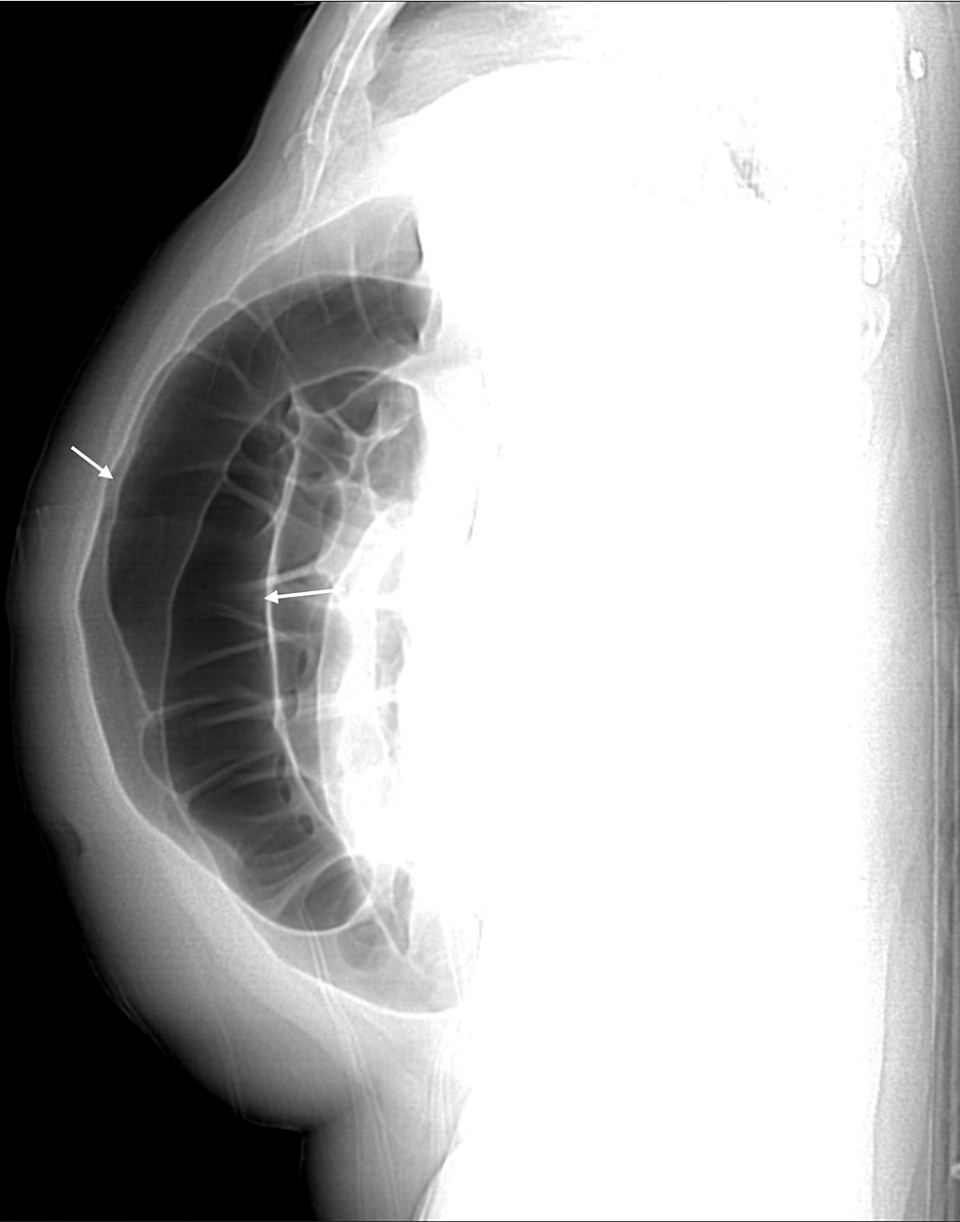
Abdominal CT in sagittal view shows severe colonic dilatation (White arrows).
